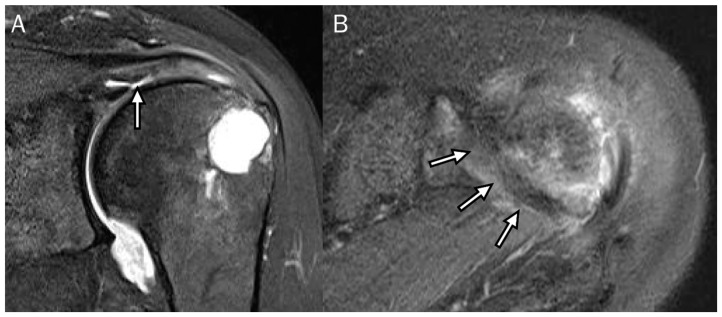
Articular-delaminated partial-thickness tear of the supraspinatus tendon with retraction (no visible cleavage). (A) Oblique coronal fat-suppressed T2-weighted image and (B) axial fat-suppressed T2-weighted image demonstrate an undersurface delaminated tear with subsequent retraction presenting as a retracted rotator cable (arrows).
